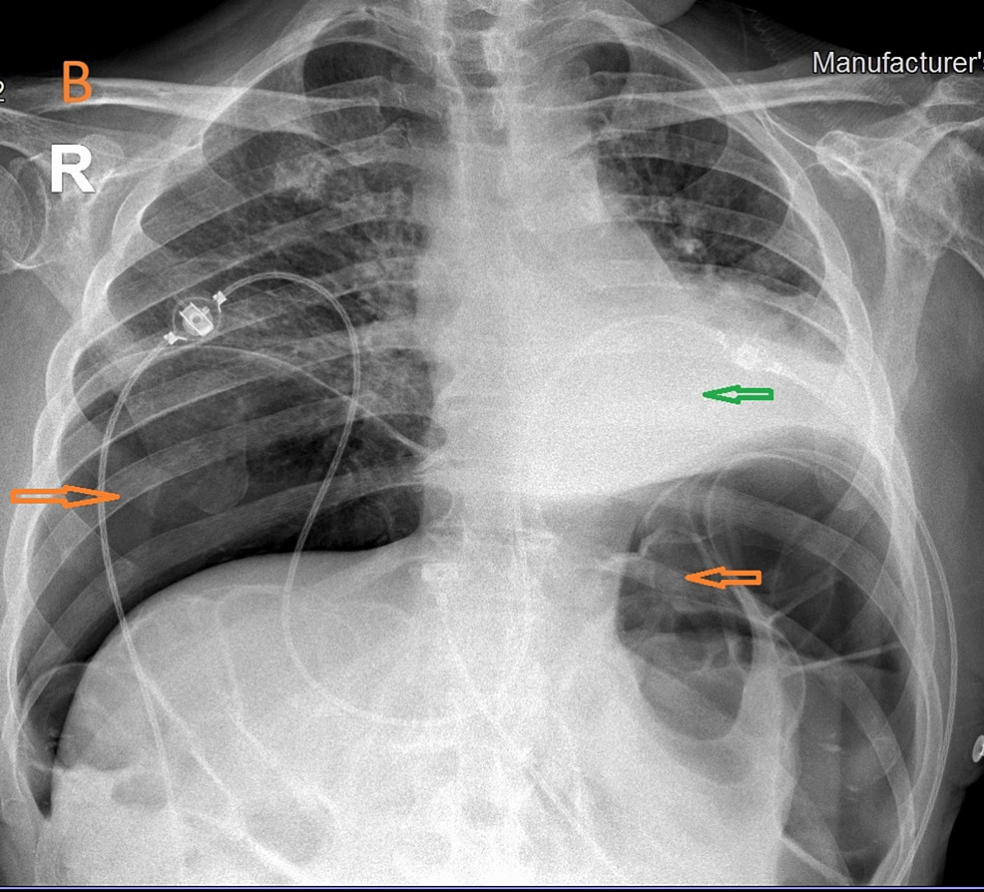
Upright Chest X-ray two days after the procedure. A large pneumoperitoneum (orange arrows) and collapse of the left lower lobe (green arrow) are observed.
